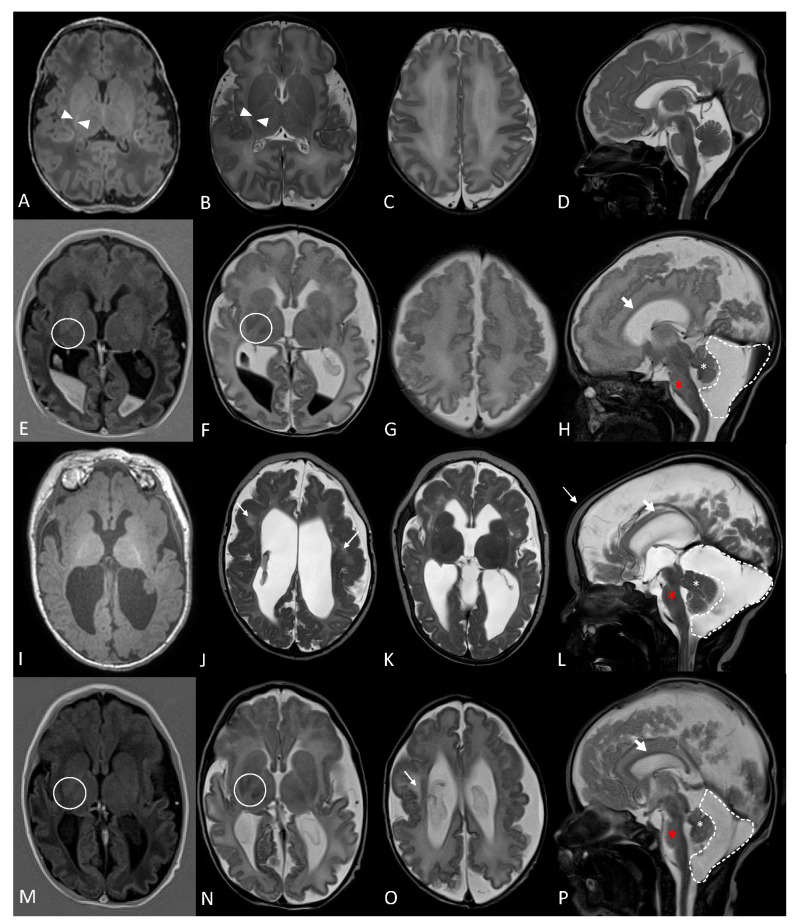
Magnetic resonance imaging of Subjects 1 and 2. (A–D) Normal MR head performed on an asymptomatic term patient with elevated bilirubin. Axial T1 (A), Axial T2 (B,C), and Sagittal T2 (D) images show the expected normal T1 hyperintense, T2 hypointense myelin signal within the posterior limb of the posterior capsule (A,B, white arrowhead) with a normal gyration pattern and midline structures, including the corpus callosum, brainstem, and cerebellum. (E–H) Subject 1 at 11 days of age. Axial T1IR (E), Axial T2 (F,G), and Sagittal T2 (H) images show the absence of the normal myelin signal within the posterior limb of the internal capsule (E,F, Circle) and extensive polymicrogyria with frontoparietal predominance. Brain atrophy noted with secondary enlargement of the lateral ventricles. Intraventricular hemorrhage is present. The corpus callosum is thinned (H, thick white arrow). The superior cerebellar vermis is dysplastic (H, white asterisk), and the pons are hypoplastic (H, red asterisk). A large mega cisterna magna is also noted (H, dashed line), containing some blood. (I–L) Subject 1 at 4 months of age. Axial T1 (I), Axial T2 (J,K), and Sagittal T2 (L) images show interval progression of myelination and development of white matter changes (J,K), thin white arrow). (M–P) Subject 2 at 8 days of age. Axial T1IR (M), Axial T2 (N,O), and Sagittal T2 (P) images show the absence of the normal myelin signal within the posterior limb of the internal capsule (M,N, Circle), extensive polymicrogyria with frontoparietal predominance, and patchy white matter changes. Brain atrophy noted with secondary enlargement of the lateral ventricles. The corpus callosum is thinned (P, thick white arrow). The superior cerebellar vermis is dysplastic (P, white asterisk), and the brainstem, specifically the pons, is hypoplastic (P, red asterisk). A large mega cisterna magna is also noted (P, dashed line).
